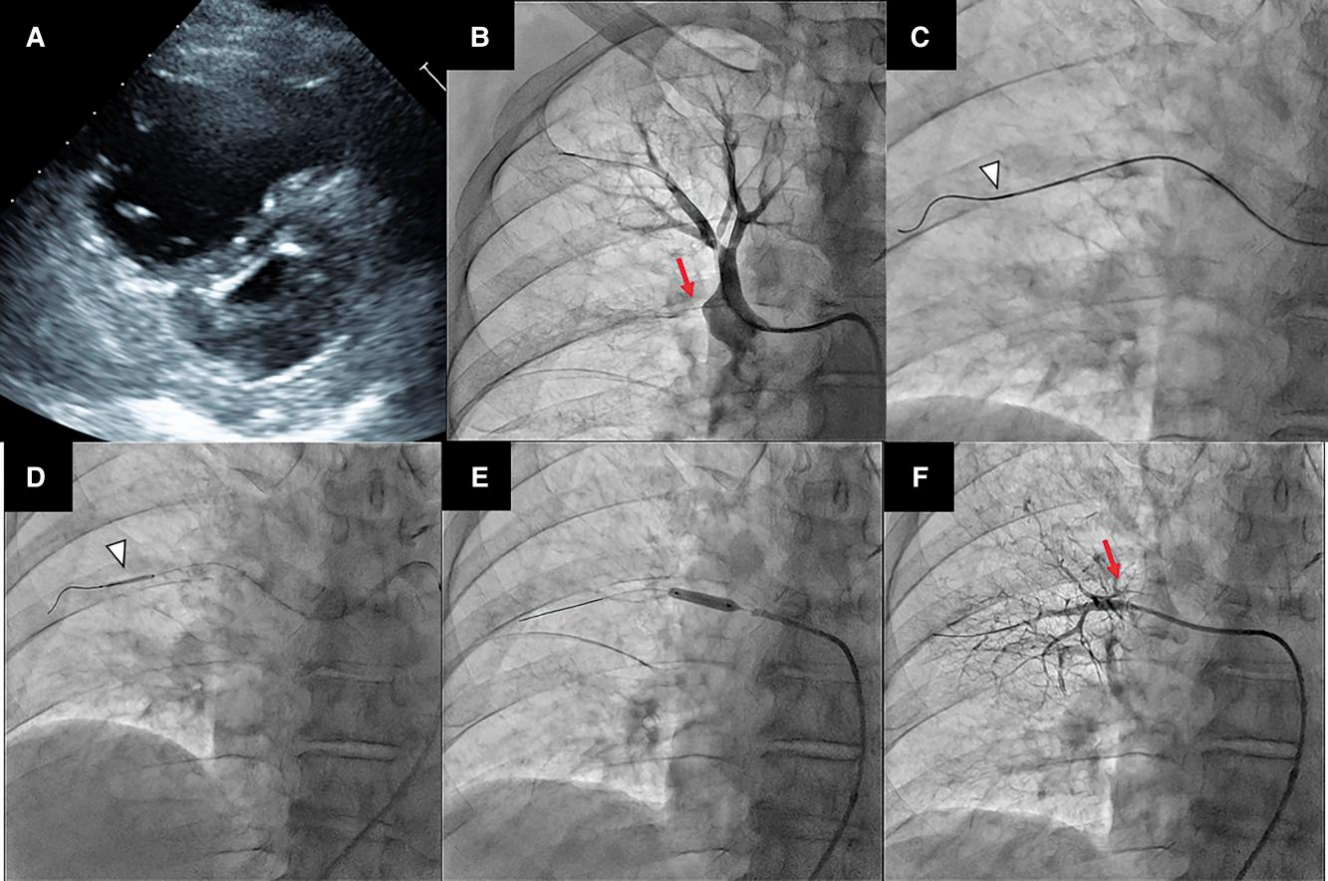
(*A*) Echocardiography revealed a flattened interventricular septum. (*B*) Pulmonary angiography revealed total occlusion lesion in the A3 segment (arrow). (*C*) Corsair enabled passage to total occlusion lesion with the rotational bougie technique (arrowhead). (*D*) Passage and dilation of a 2 mm balloon (arrowhead). (*E*) Passage and dilation of a 4 mm balloon. (*F*) Pulmonary angiography after the procedure revealed good reperfusion (arrow) and pulmonary venous return.
